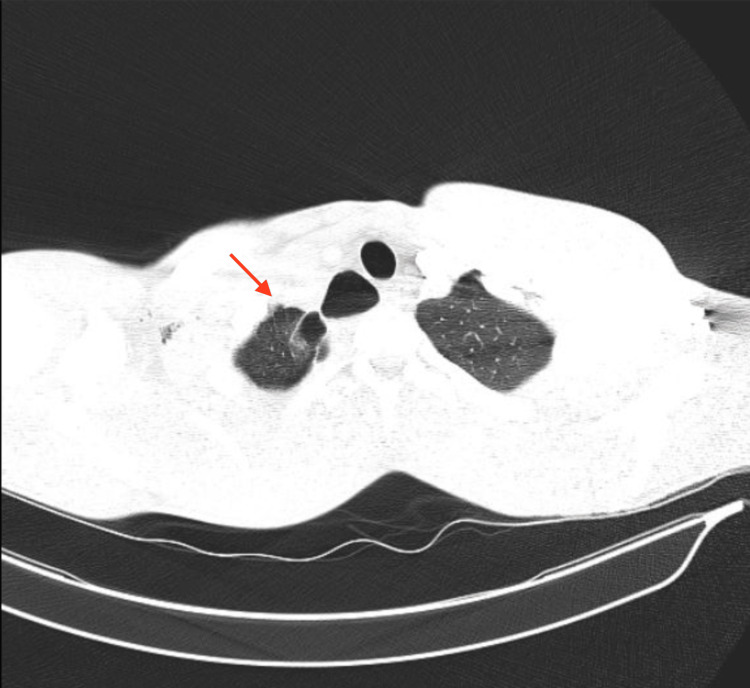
CT thorax with contrast: multiple right apical blebs and residual right pneumothorax
